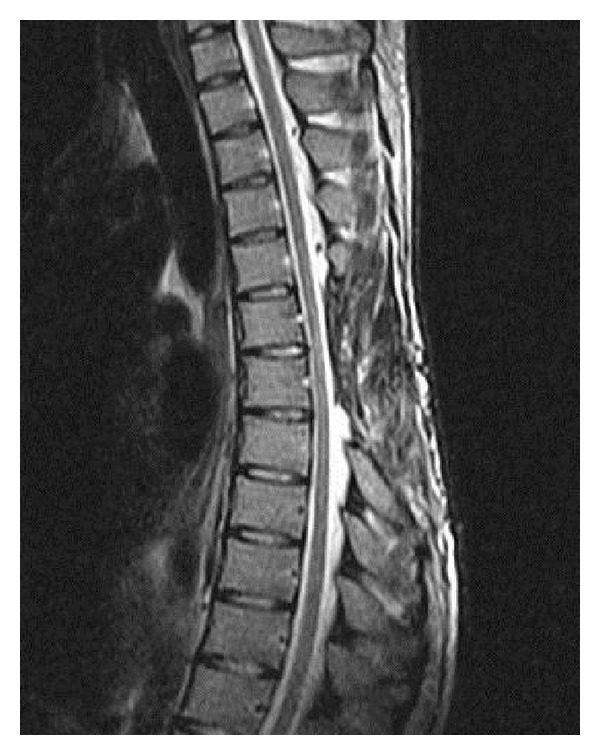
Postoperative 2-year follow-up sagittal MRI showed no expansive mass on the spinal cord and no recurrence.
